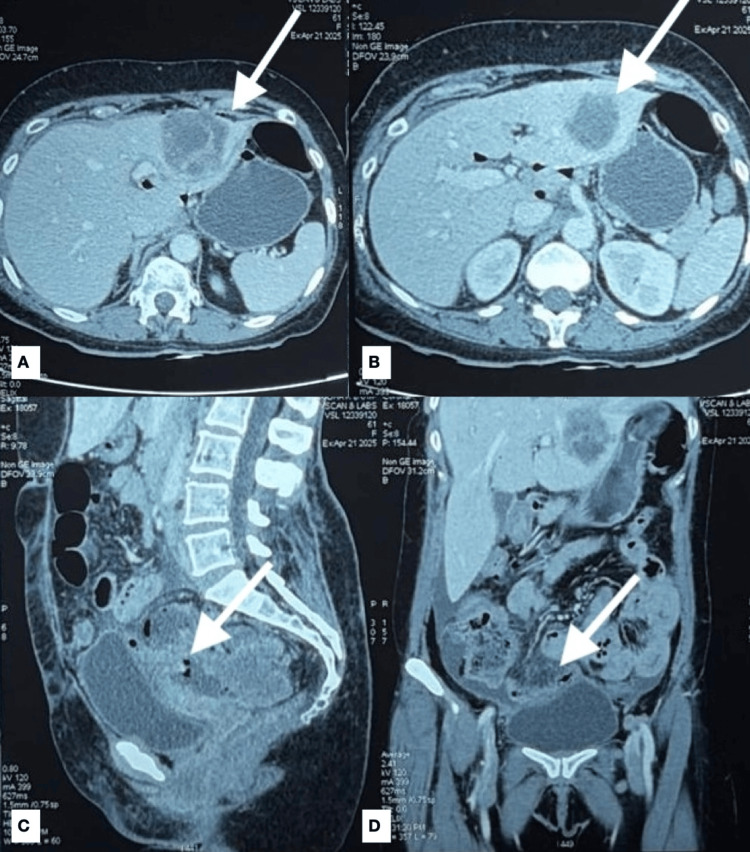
Preoperative contrast enhanced computed tomography of the abdomen A, B) Contrast-enhanced computed tomography of the abdomen in axial view showing a solitary large hepatic lesion occupying the left lobe of the liver, suggestive of metastatic disease; C) Contrast-enhanced computed tomography of the abdomen in Sagittal view showing a right adnexal mass lesion with localized air foci surrounding it - suggestive of pneumoperitoneum; D) Contrast-enhanced computed tomography of the abdomen in coronal view showing air foci in the pelvis surrounding the adnexal mass lesion, suggestive of pneumoperitoneum.
